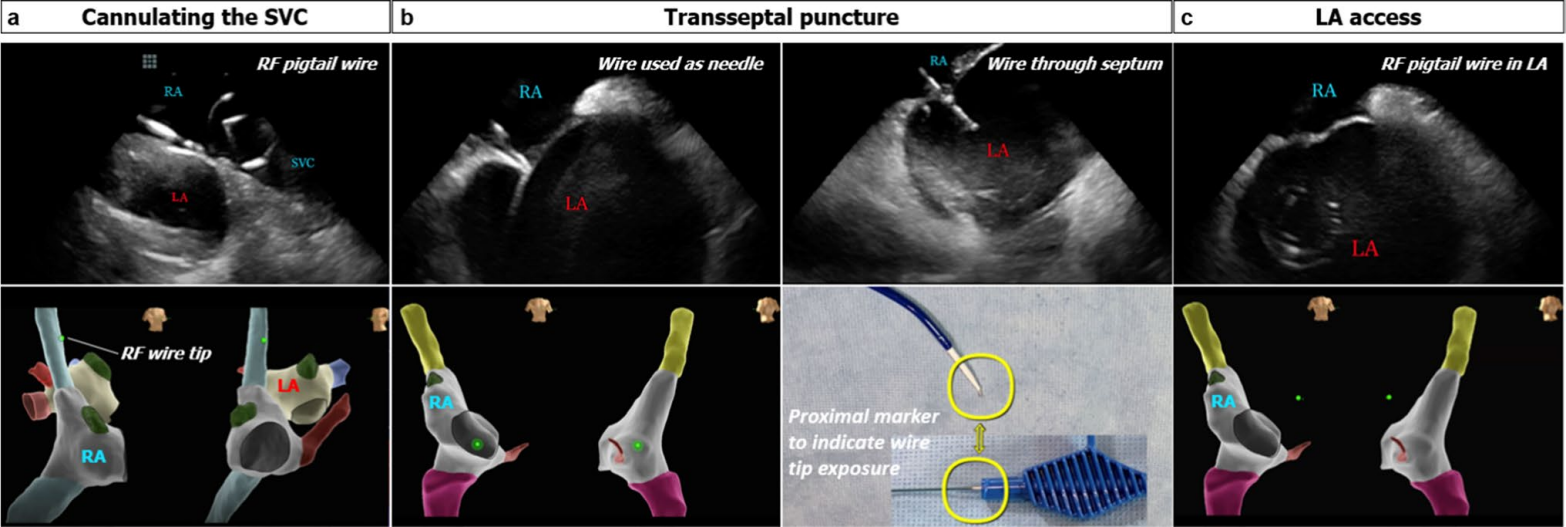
Visualization of RF wire with intracardiac echocardiography and electroanatomic mapping during (a) SVC cannulation, (b) transseptal puncture, and (c) LA access. Top panels: intracardiac echocardiography visualization of RF pigtail wire in SVC, RF wire tenting interatrial septum, RF wire through septum after RF energy application, and RF pigtail wire in LA. Bottom panels: electroanatomic visualization of RF wire tip in SVC, in the RA, and in the LA. SVC, superior vena cava; RA, right atrium; LA, left atrium
